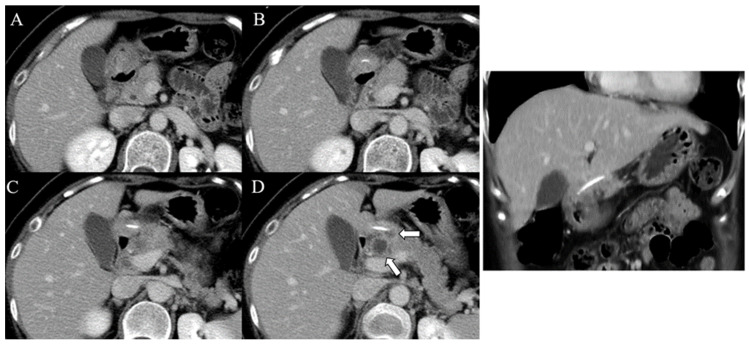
Contrast-enhanced CT imaging with serial sections in the axial (A–D) and coronal planes displaying a linear and hyperdense foreign body penetrating through the posterior wall of the gastric antrum into the pancreatic head. The white arrows indicate abscess formation in the pancreatic head and near the ingested foreign body.
